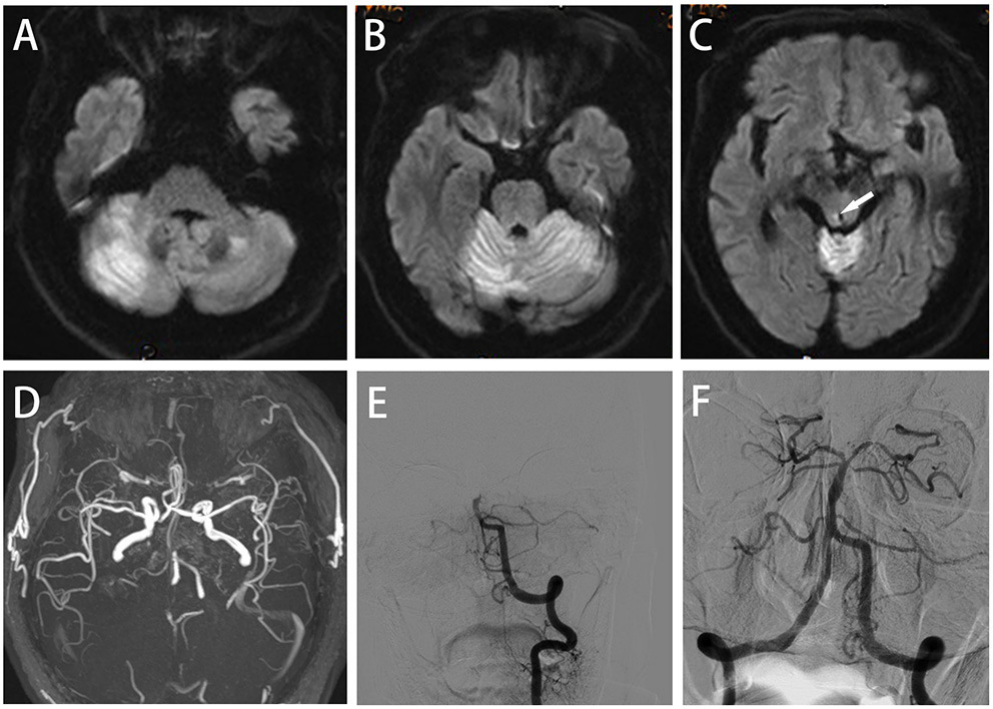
Case 3: (A–C) Axial diffusion-weighted imaging (DWI) showing acute cerebral infarction in the bilateral cerebellar hemispheres, cerebellar vermis and periaqueductal gray (arrowheads). (D,E) Magnetic resonance angiography (MRA) and AP left VA angiography demonstrating basilar artery occlusion; successful recanalization was obtained by endovascular thrombectomy (F).
